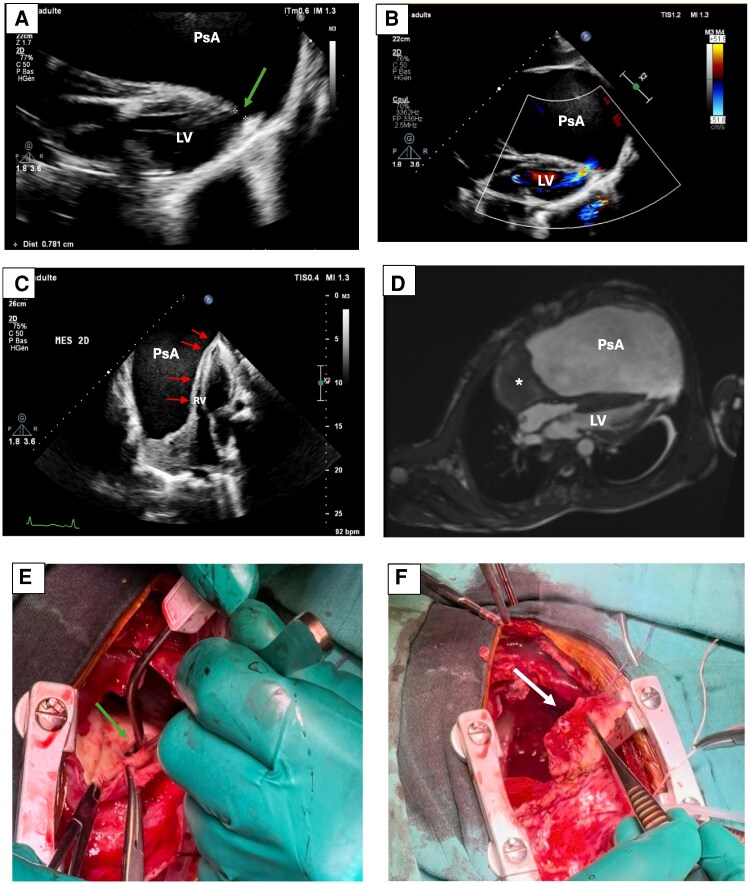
Multimodal assessment of a giant left ventricular pseudoaneurysm. (*A*) Transthoracic echocardiography (subcostal view) showing the left ventricular pseudoaneurysm, with the neck measuring 8 mm indicated by the green arrow. (*B*) Transthoracic echocardiography, Color Doppler demonstrating a systolic–diastolic flow across the neck. (*C*) Transthoracic echocardiography, four-chamber view, showing right heart chamber collapse caused by a left ventricular apical pseudoaneurysm. The red arrows indicate the significant mechanical compression of the right ventricle free wall, highlighting the mass effect exerted by the giant sac. (*D*) Cardiac magnetic resonance imaging (cine T1 sequence, four-chamber view) showing a partially thrombosed (asterisk) apical left ventricular pseudoaneurysm. (*E*) Intraoperative view showing the neck connecting the pseudoaneurysm (green arrow). (*F*) Intraoperative view showing the thickened pericardium indicated by the white arrow (pericardectomy). (Moving image available in the online version of the article.)
